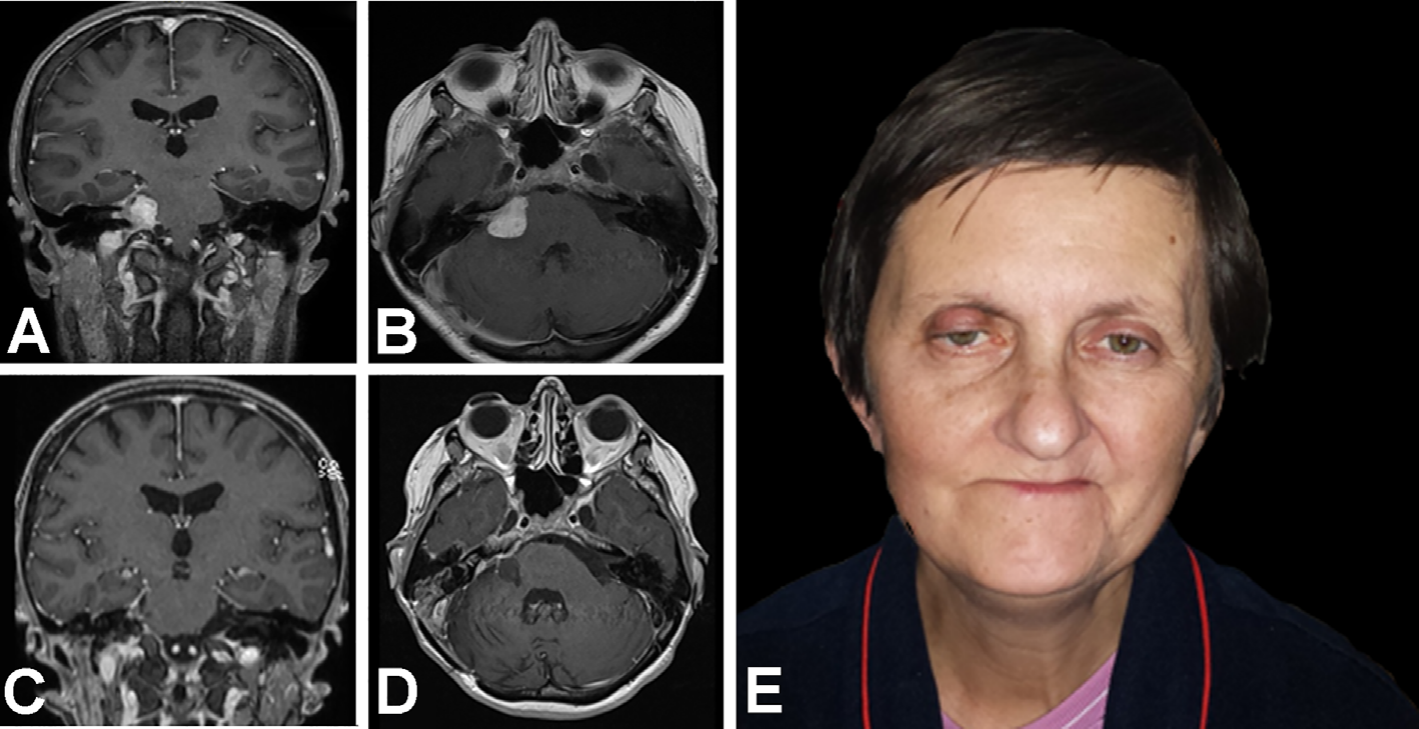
Magnetic resonance T1WI contrast enhanced images and photograph show treatment results in a 54–year old woman with facial nerve paresis after a subtotal resection of a vestibular schwannoma. At one year after the initial surgery, (A) coronal and (B) axial MR images show growth after stereotactic radiosurgery. Then, revision surgery was performed, *via* a retrosigmoid approach with facial neurorrhaphy; 11 months later, (C) coronal and (D) axial T1WI MR images show the results of a gross total resection with (E) satisfactory facial nerve function.
